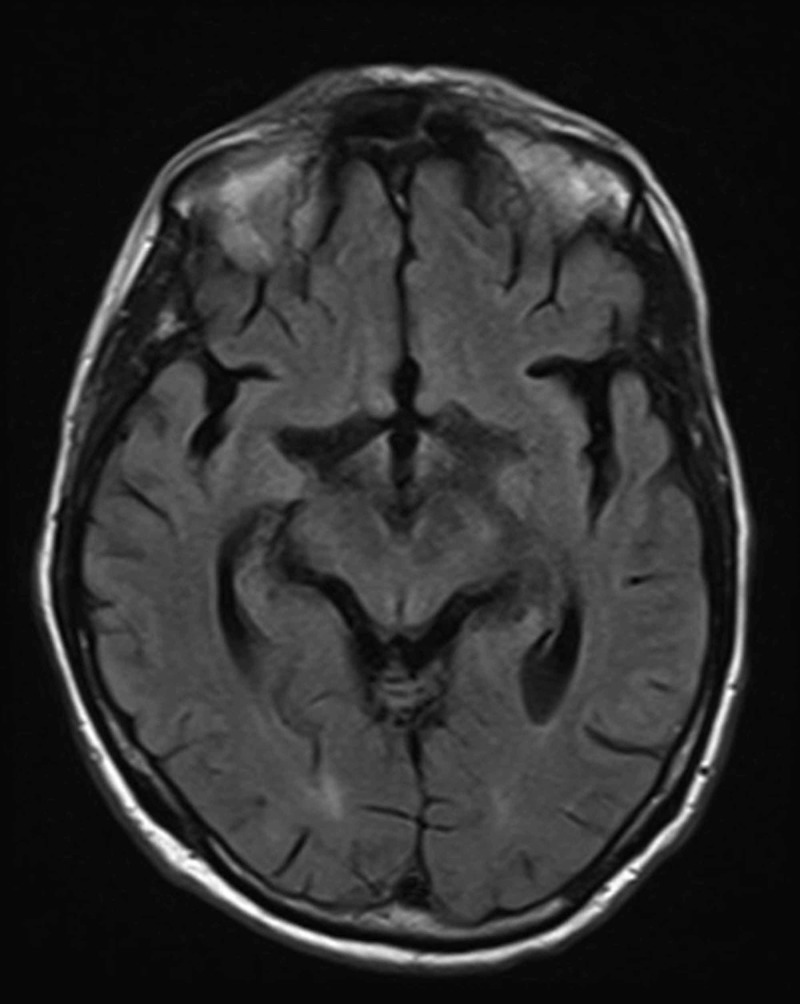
T2-weighted MRI of brain. T2-weighted MRI of brain revealing no acute intracranial pathology.
